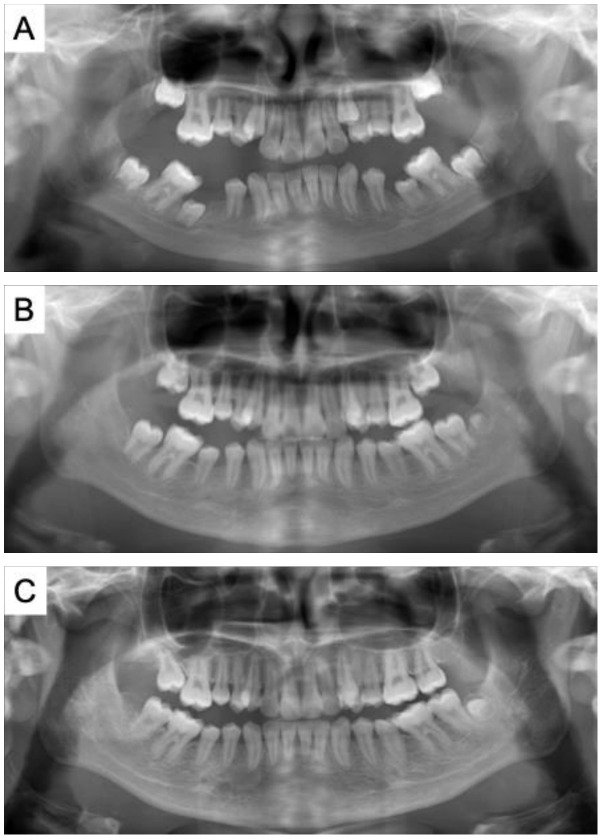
Longitudinal panoramic radiographs showing the increased thickness of the alveolar bone in the molar region. (A) 10 years and 10 months, (B) 12 years and 8 months, (C) 14 years and 8 months.
